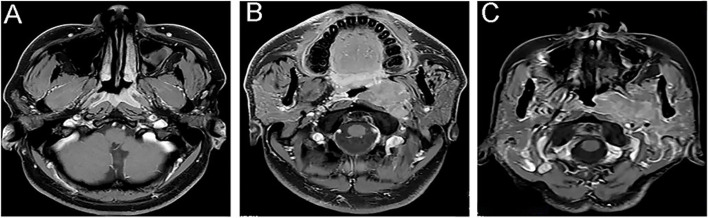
MRI findings of the case 1, 2, 3. MRI showed the thickening area of the roof posterior and bilateral walls of the nasopharyngeal, mainly involved in the left (case 1, A). MRI showed a mass involved in the area of roof posterior and left walls, with heterogenous enhancement, and the lesion size was about 35 mm × 44 mm × 65 mm (case 2, B). MRI showed the thickening area of the roof posterior and bilateral walls of the nasopharyngeal, mainly involved in the left (case 3, C)
